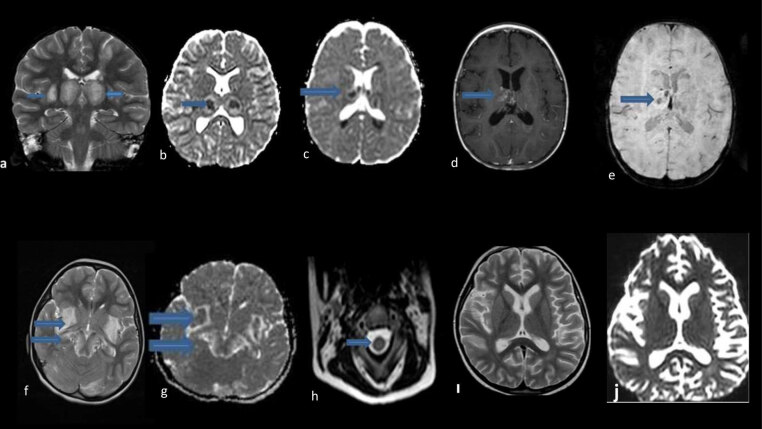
MRI images of our cases. a: in the coronal T2-weighted image of case 1, swelling and hyperintensity in the bilateral thalamic and external capsules and hyperintensity in the left parietal lobe; b: bilateral restriction is seen in the apparent diffusion coefficient (ADC) of the same patient; c: in the ADC of case 2 bilateral thalami and periventricular white matter restriction; d–e: postcontrast T1-weighted images of the same patient reveal more pronounced contrast enhancement pattern in the right thalamus and Hemosiderin deposition is observed in the left thalamus in susceptibility weighted imaging (SWI); f–j: case 5. (f) axial T2-weighted images demonstrating bilateral brainstem, medial temporal lobe, external capsule, occipital involvement, (g) markedly restriction in lesions described in ADC, (h) axial T2-weighted cervical images shows C4-C6 hyperintensity, (ı–j) axial T2-weighted images demonstrating and the apparent diffusion coefficient (ADC) control MRI lesions were seen to regress almost completely after treatment.
